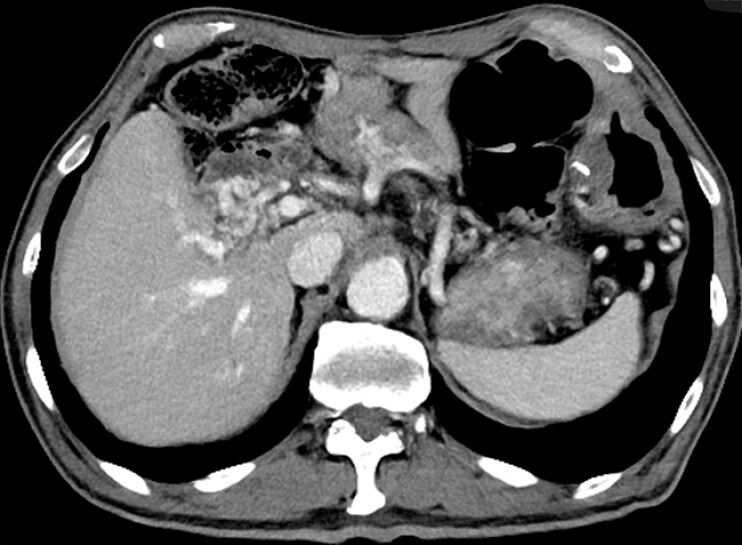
Abdominal computed tomography image showing worsening stenosis of the superior mesenteric vein due to tumor growth and the development of collateral blood vessels around the bile duct–jejunal anastomosis.
